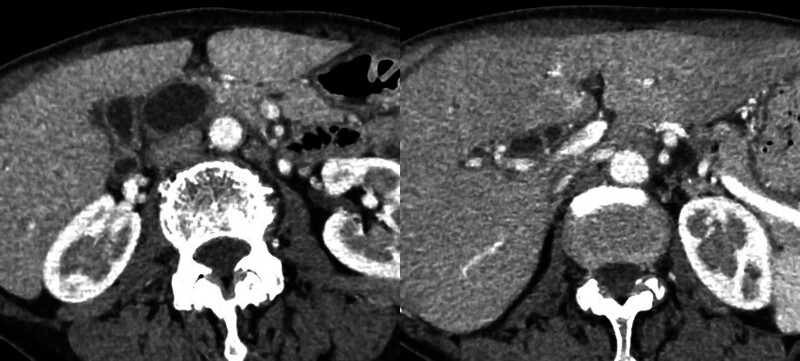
Contrast-enhanced computed tomography scan showing dilatation of the common bile duct without dilatation of the intrahepatic bile duct and diffused wall thickening of the bile duct and gallbladder walls.
